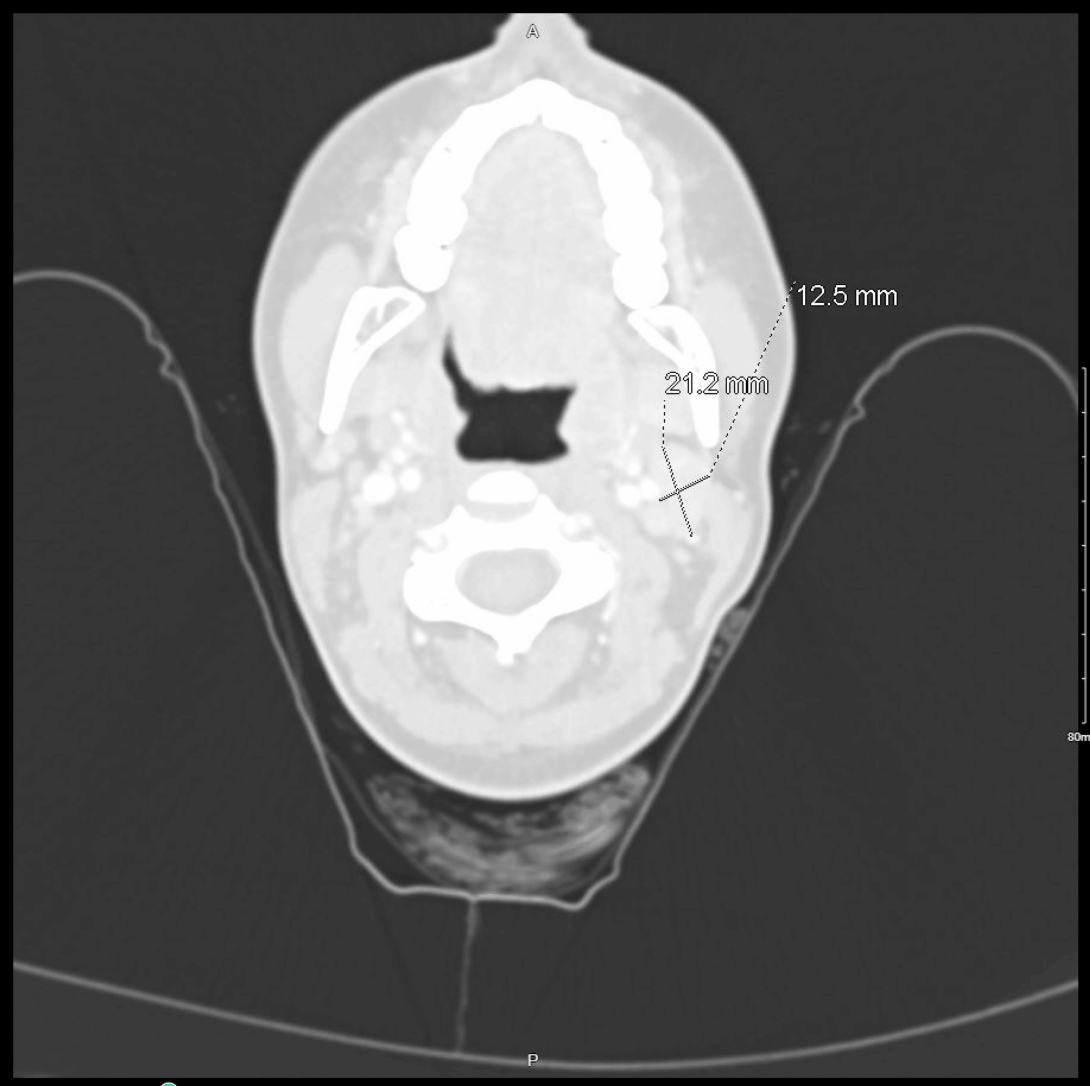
CT of the soft tissue of the head and neck with contrast revealing a borderline enlarged left cervical lymph node measuring 2.1×1.2 cm CT: computed tomography
